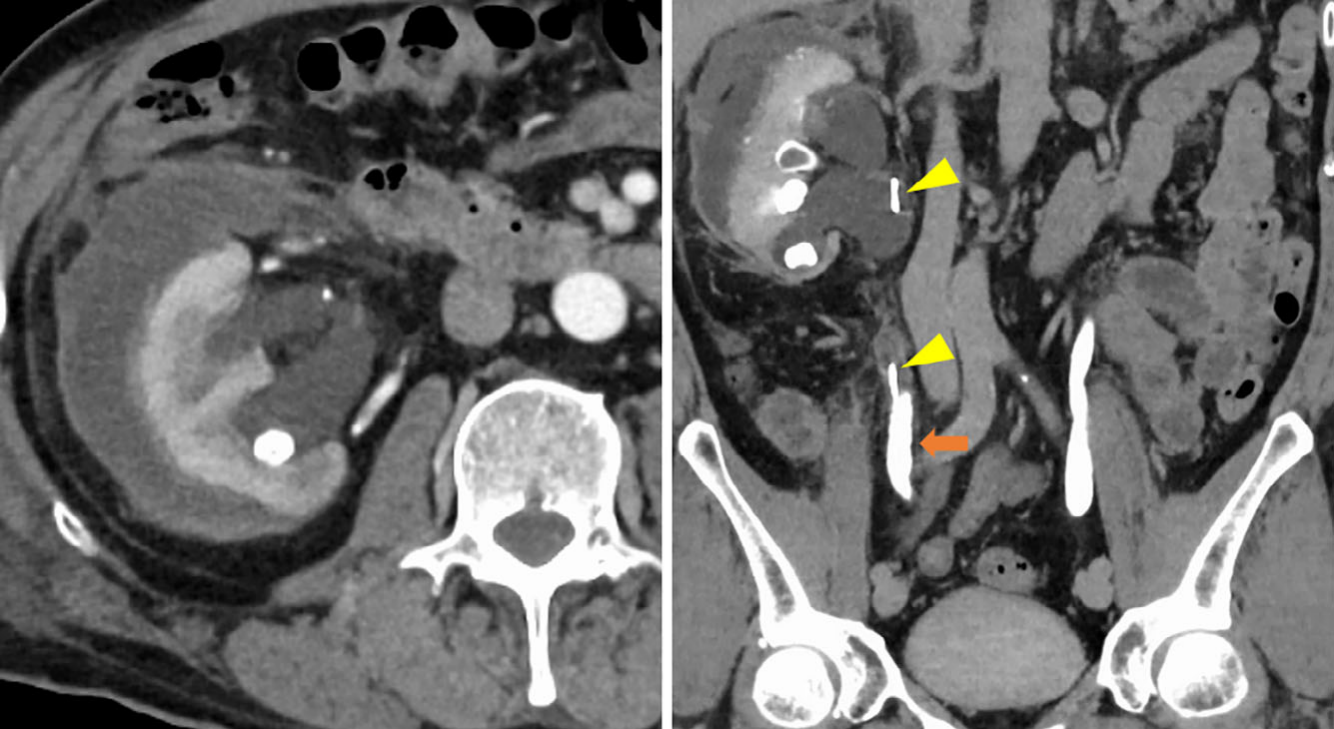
CT showing right hydronephrosis and a subcapsular renal hematoma (arrow: stone street, arrowhead: ureteral stent). [Colour figure can be viewed at wileyonlinelibrary.com]
